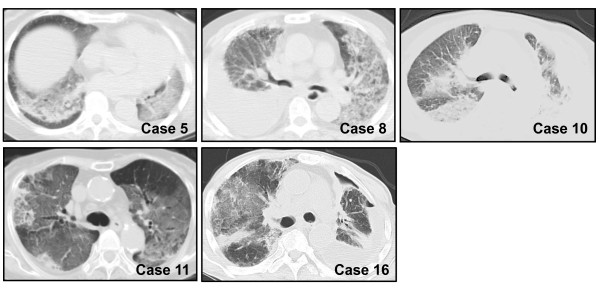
Chest CT images of five patients who developed EGFR-TKI induced acute interstitial pneumonia (AIP). Representative chest CT images of the five patients who developed AIP pattern of EGFR-TKIs induced ILD are shown. Each case number corresponds to the patient's number listed in Table 3.
